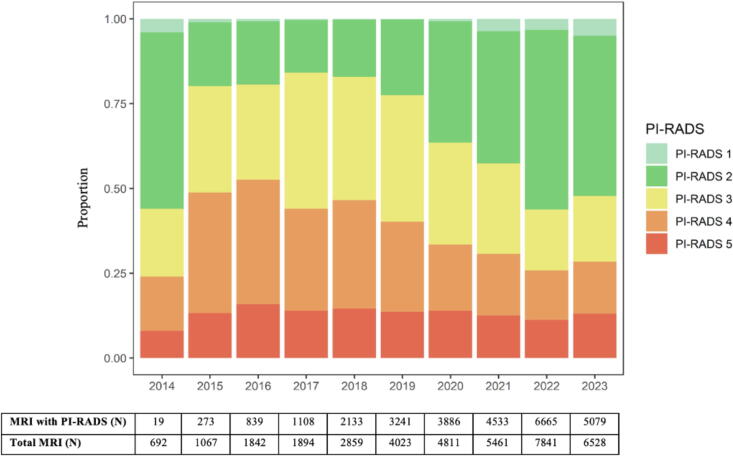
Distribution of PI-RADS scores among men ≥40 years with PSA ≥3 ng/ml undergoing MRI without a prior prostate cancer diagnosis from 2014–2023.
